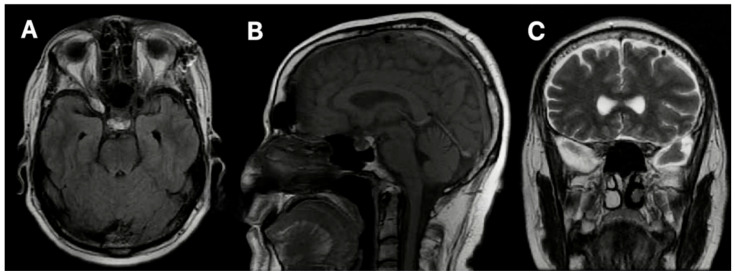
Case 1’s postoperative MRI shows no residual tumor but mild frontal pneumocephalus, which is better appreciated on the sagittal view. (A) Axial view. (B) Sagittal view. (C) Coronal view.
